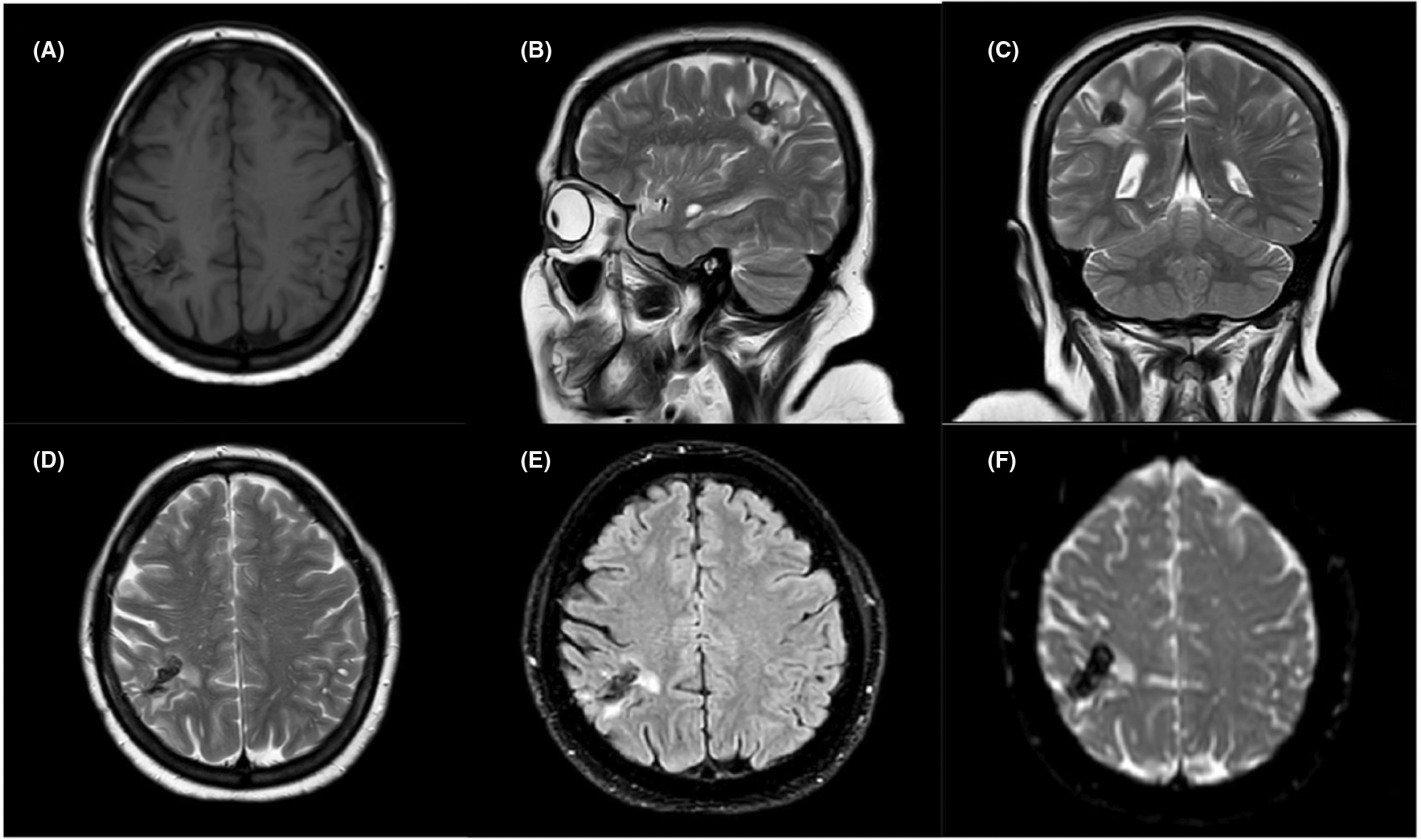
Encephalomalacia region with peripheral gliosis at the right parietal lobe due to previous surgery. (A) Axial T1‐Weighted, (B) Sagittal T2‐Weighted, (C) Coronal T2‐Weighted, (D) Axial T2‐Weighted, (E) Axial T2‐weighted‐Fluid‐Attenuated Inversion Recovery (FLAIR), (F) Diffusion‐weighted MRI (DWI)
